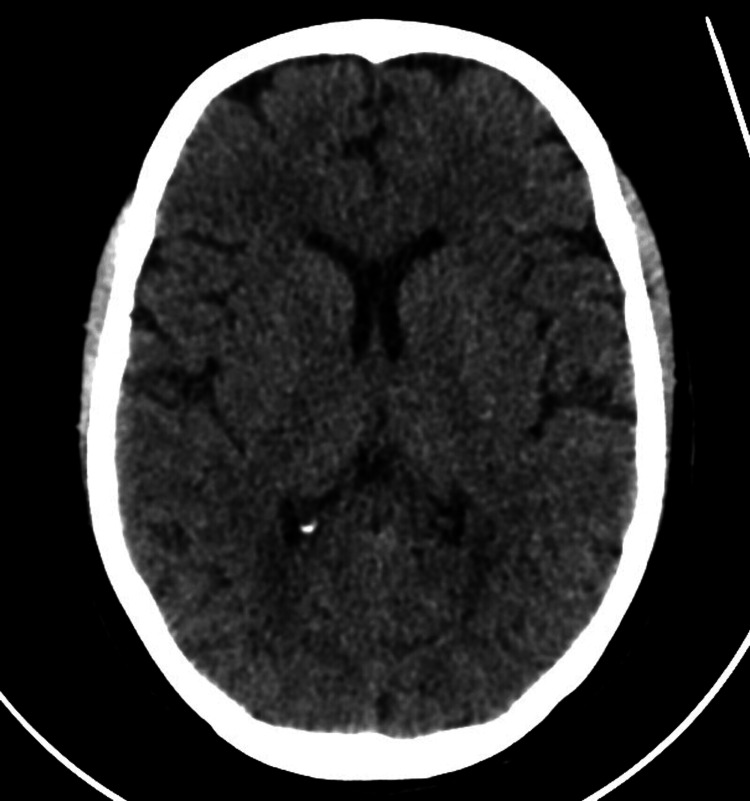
CT head axial view with no new intracranial bleed or space-occupying lesion.
